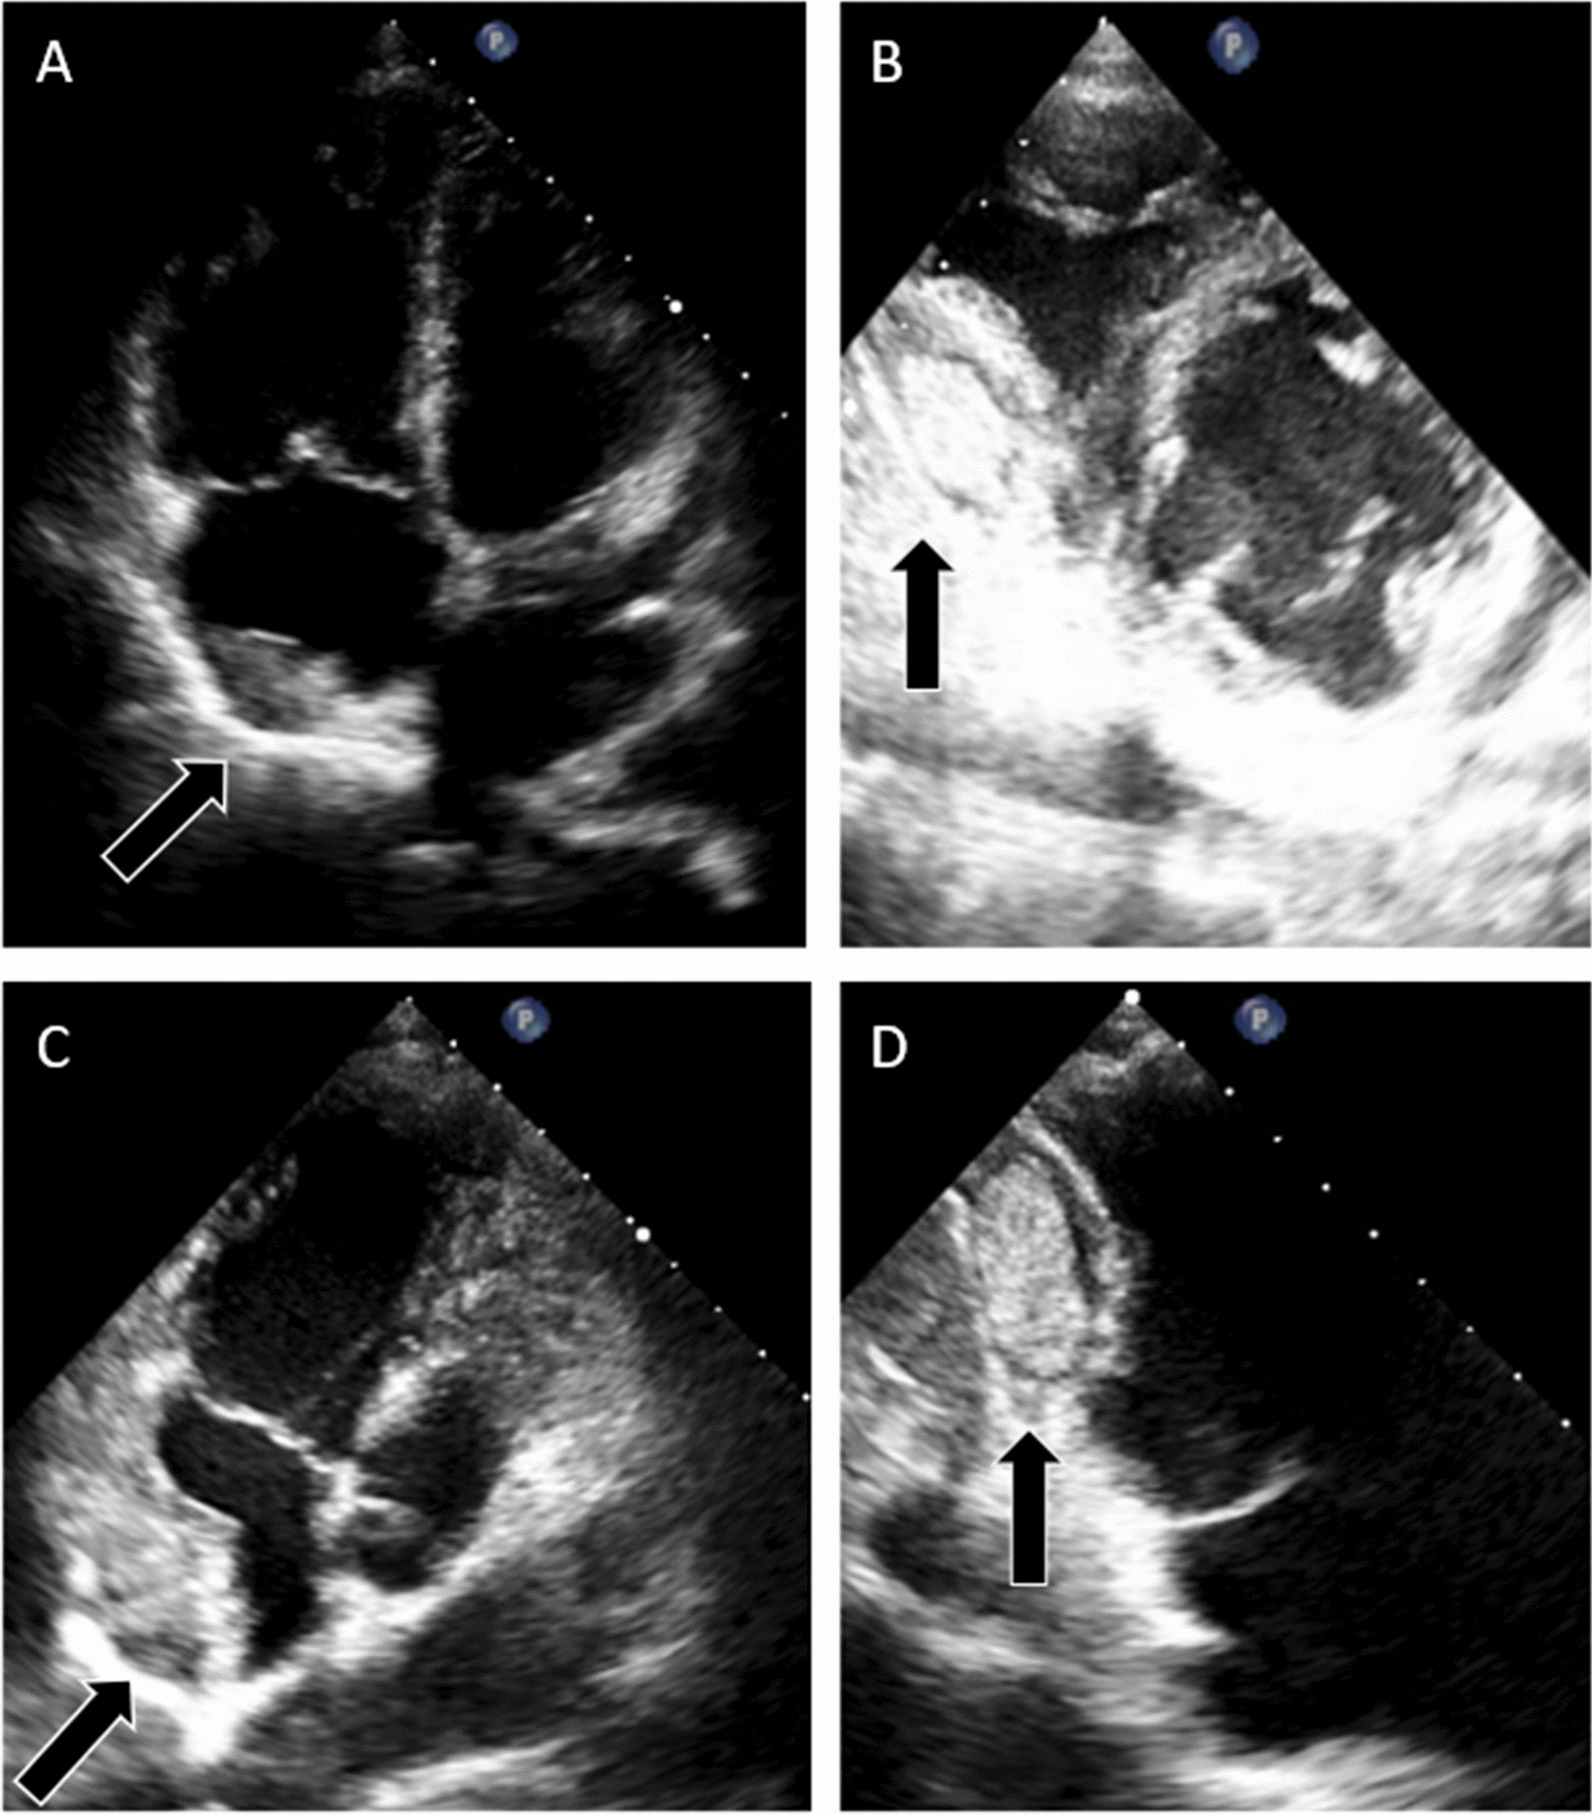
Transthoracic echocardiogram. The mass (Black Arrow) occupied the pericardial space next to the apical four-chambers view (a). The parasternal short-axis view (b) and Apical RA-focused view (c) revealed an indistinct border mass around the RA side. Apical RV-focused view (d) showed the mass near RV located within pericardial space
